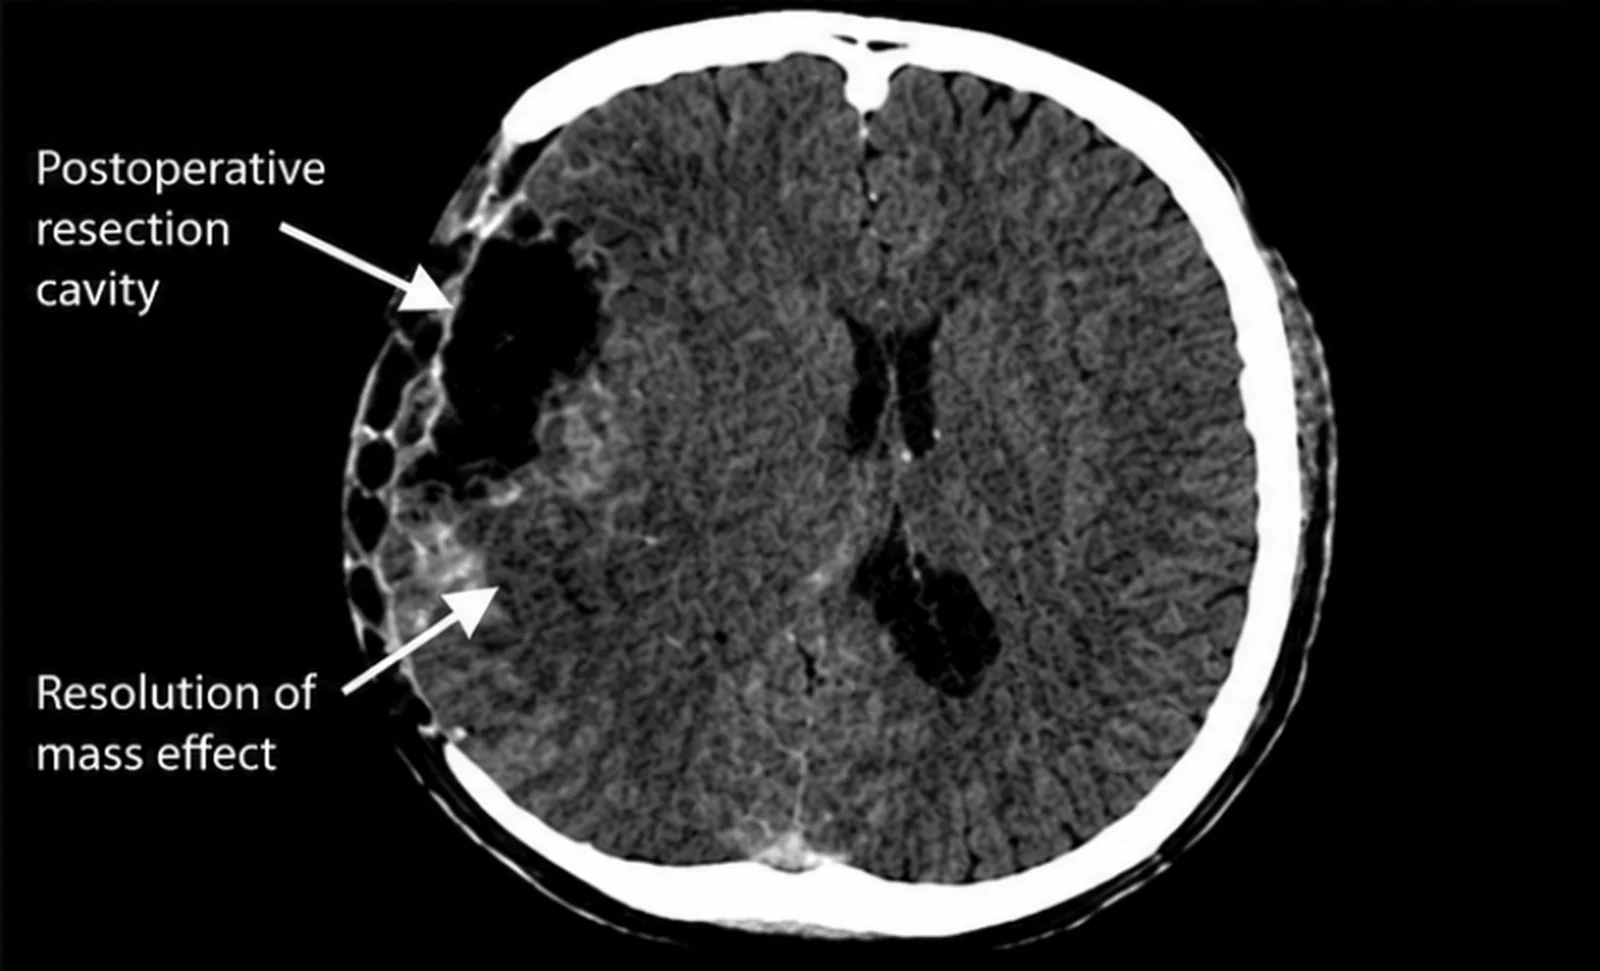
Postoperative CT scan. Postoperative axial computed tomography (CT) demonstrating the surgical resection cavity (white arrow) with marked reduction of the mass effect (arrowhead), consistent with satisfactory decompression following tumor resection.
